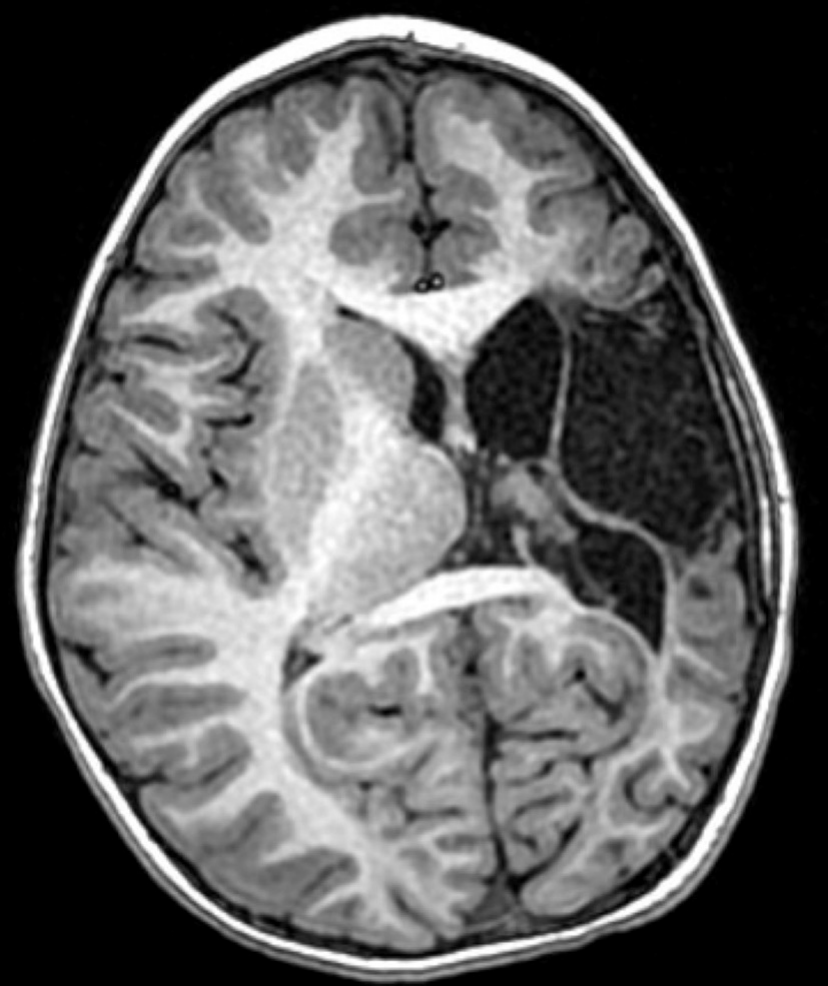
Patient #x. Axial T1-weighted MRI obtained at age 5 years, demonstrating a left middle cerebral artery distribution infarct and a disproportionately small left cerebral hemisphere.
